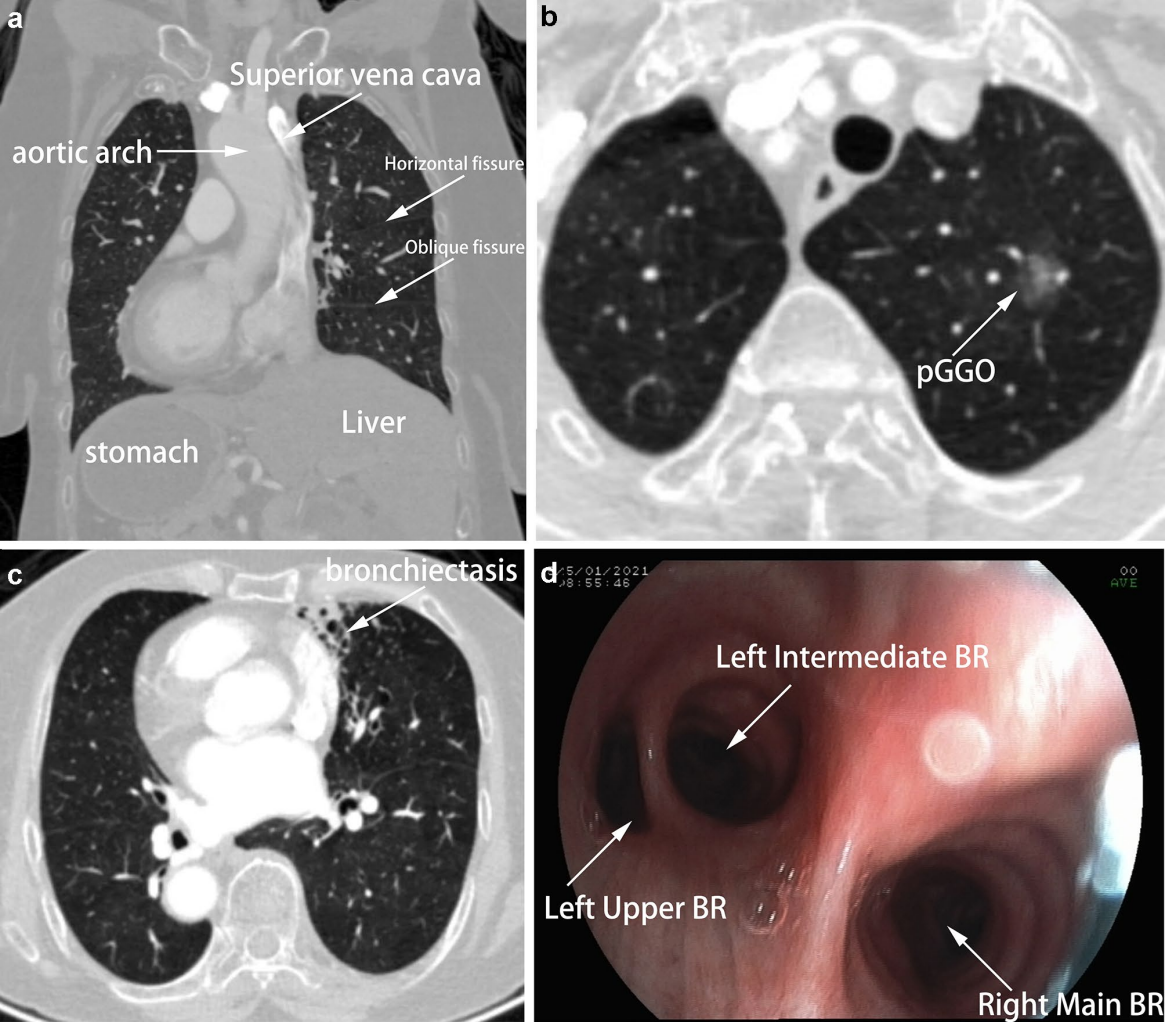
The radiological findings of the pulmonary tumor and thoracic anatomy. a Situs inversus totalis (dextrocardia, aortic arch, stomach, and liver). b Chest CT revealed a pure ground-glass opacity (pGGO) (measuring 10 × 12 mm in diameter) located in LS^1^. c Bronchiectasis with local inflammation was seen in the left middle lobe. d Bronchoscopy confirmed that the left and right bronchial branches were mirror-images of each other
